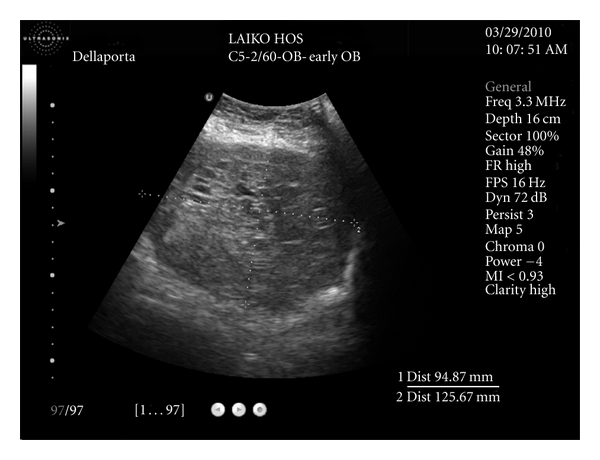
Explanatory legend: Transvaginal ultrasound of the patient. Snowstorm-like appearance. Uterus dimension: 94,87 mm × 125,67 mm.
